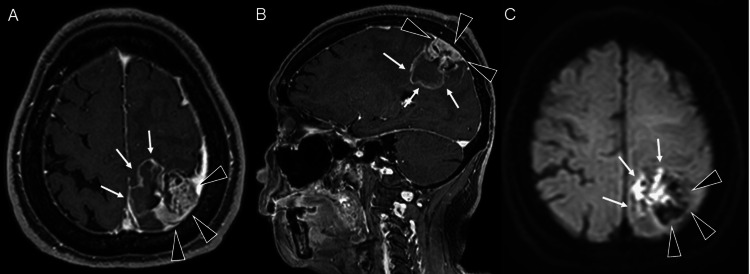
Brain MRI findings. Gadolinium-enhanced T1-weighted images showing a left parietal lobe tumor broadly attached to the dura with associated dural thickening. The dural-based solid component (A, axial; B, sagittal; black arrowheads) exhibits heterogeneous enhancement, whereas the deeper portion shows irregular morphology with marginal and poor internal enhancement, suggestive of necrosis (white arrows). On diffusion-weighted imaging (C), the dural-based solid component demonstrates low signal intensity (black arrowheads), while the deeper portion appears hyperintense (white arrows).
